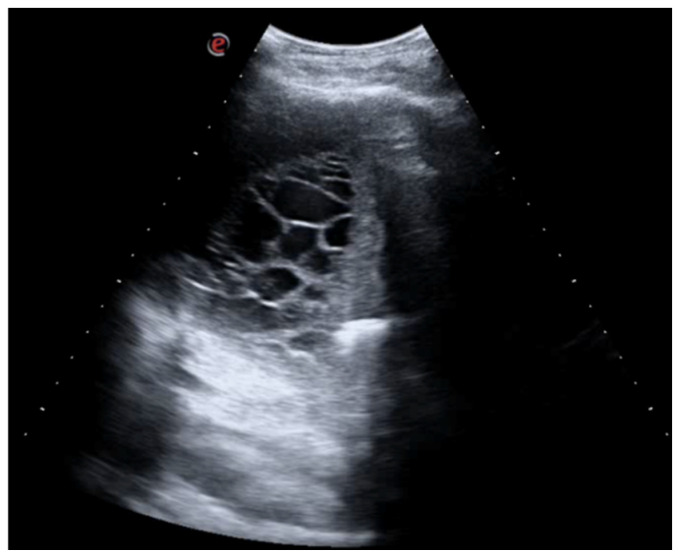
Chest ultrasound at admission showing multiloculated pleural effusion.
